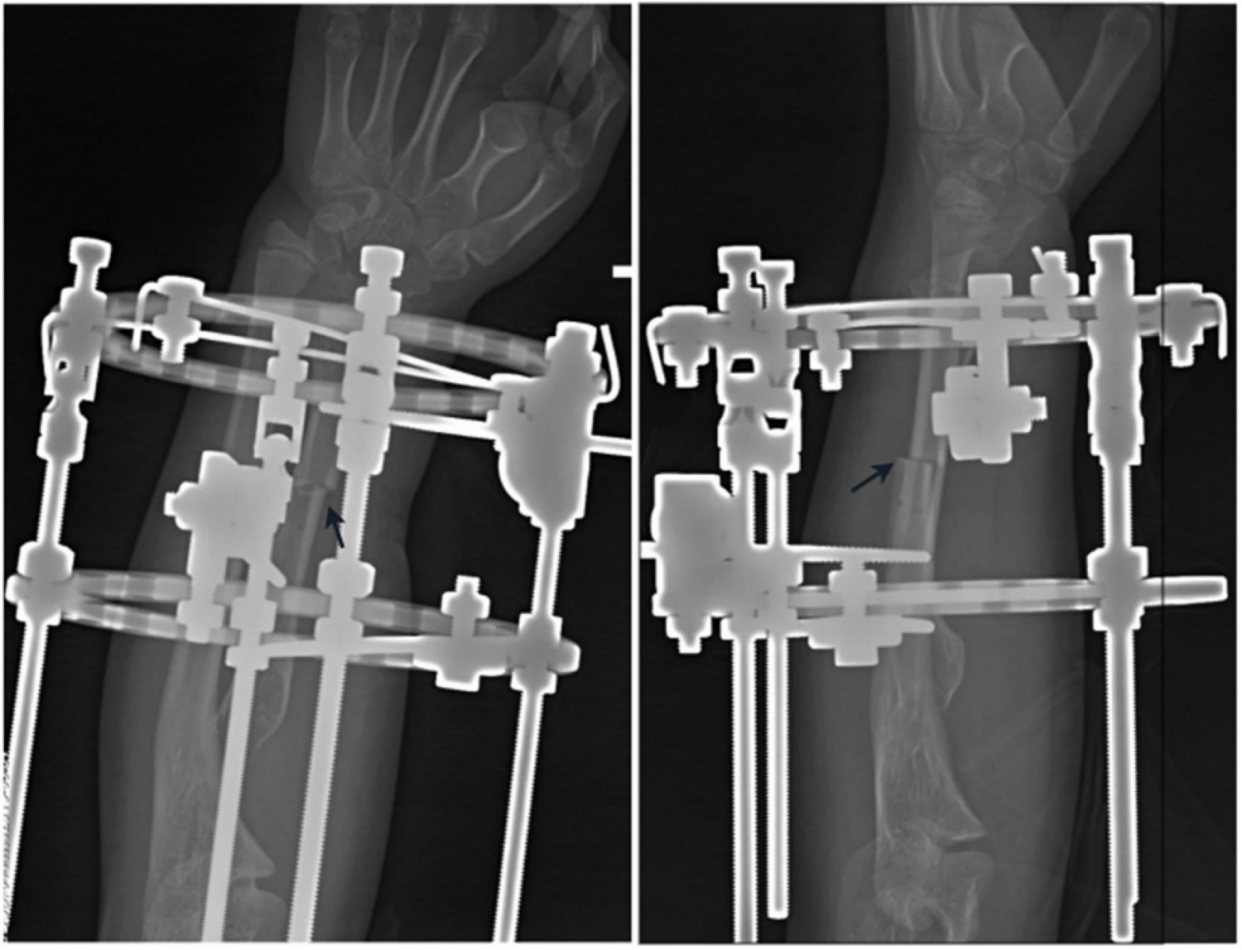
Immediate anteroposterior and lateral radiographs following radial osteotomy. The osteotomy gap(black arrow) and the fixator position are visible.
